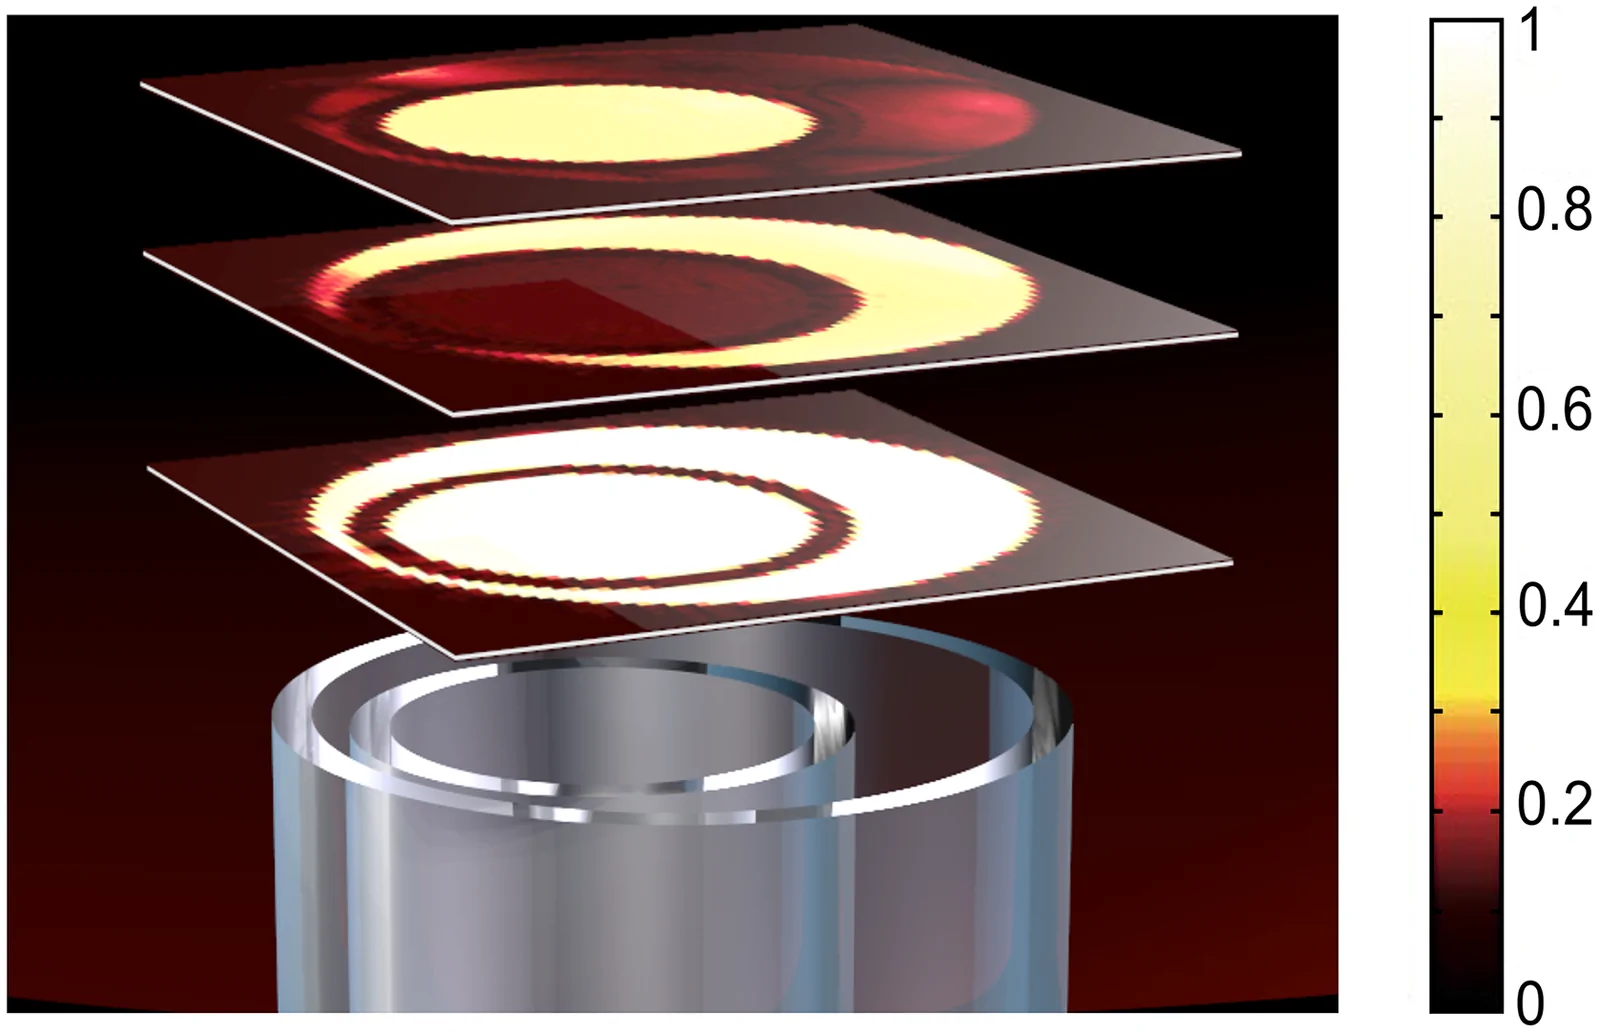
Optimal MRI contrast pulses based on different $T_{1}$ and $T_{2}$ relaxation rates. The figure shows experimental MRI microimaging results of in vitro tests of optimized imaging contrast pulses based on both $T_{1}$ and $T_{2}$ rates (inner volume: $T_{1}=$ 1800 ms, $T_{2}$ = 260 ms; outer volume: $T_{1}$ = 1400 ms, $T_{2}$ = 60 ms). Adapted from (*130*) with permission, Springer Nature. The color scale shows the pixel intensity relative to the maximum intensity of the reference image without contrast (shown at the bottom).
